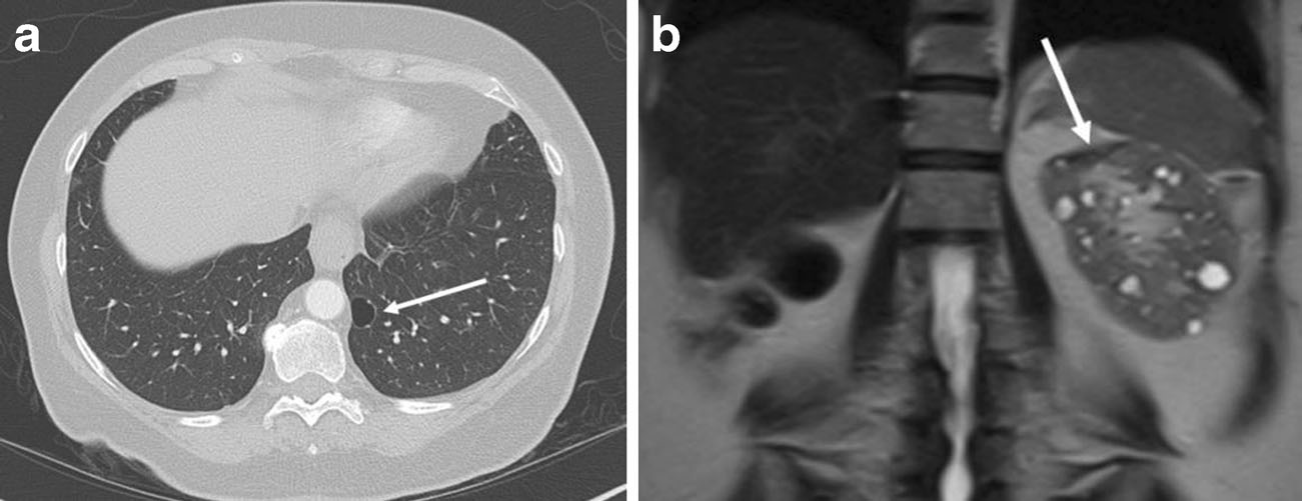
A 65-year-old woman with Birt-Hogg-Dube syndrome (previous history of RCC and oncocytomas). a The axial chest CT shows a pulmonary cyst in the left lung base (*arrow*). b The coronal single shot FSE T2-weighted image demonstrates multiple renal cysts. The *arrow* depicts post-surgical change of previous partial nephrectomy
